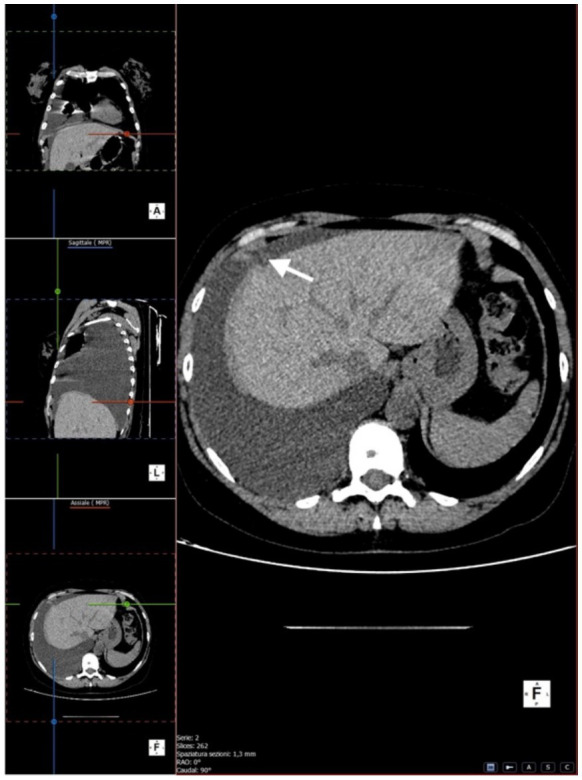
Axial CT images showing the same region of diaphragmatic irregularity (white arrow).
